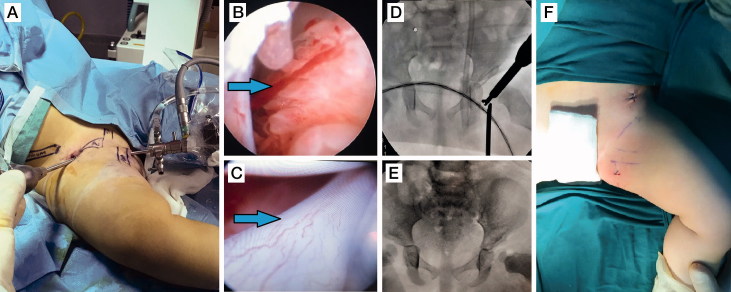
Arthroscopic technique demonstrating the portals (A), intra-articular pulvinar (B), hypertrophic ligamentum teres (C), intraoperative fluoroscopic image during incision of the transverse acetabular ligament (D), and intraoperative radiographic control for concentric reduction (E).
